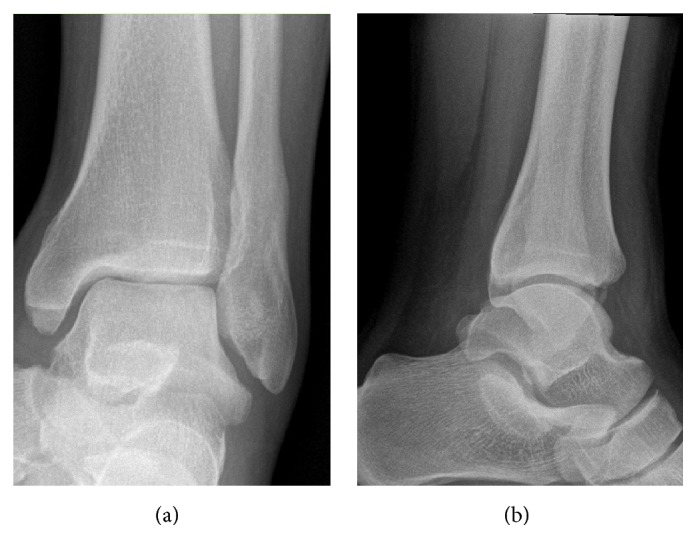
Plan (a) anteroposterior and (b) lateral radiographs of the left ankle showing good signs of healing of the lateral malleolus fracture following completing the physiotherapy rehabilitation.
